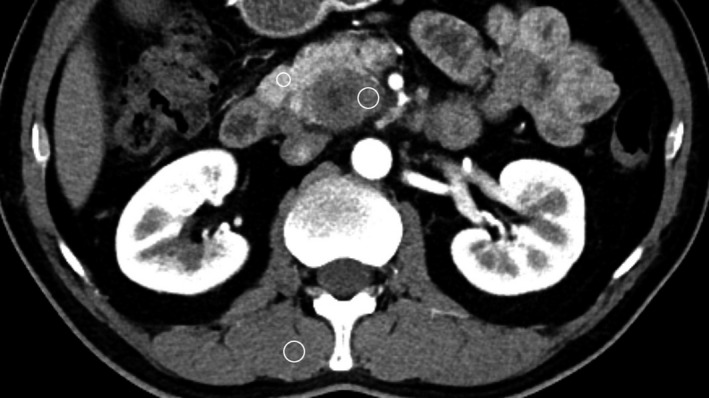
The axial enhancement computed tomography image shows a focal lesion in the pancreatic head that presented as a decreased enhanced area during the pancreatic phase. A spherical region of interest (ROI) was placed in the enhanced part of the lesion, avoiding suspected necrotic regions. A spherical ROI was placed in the adjacent pancreatic parenchyma within 10 millimeters of the lesion, avoiding the pancreatic lesion and pancreatic duct, and the partial volume was averaged for the extrapancreatic structures. A spherical ROI was placed in the erector spinae muscle
